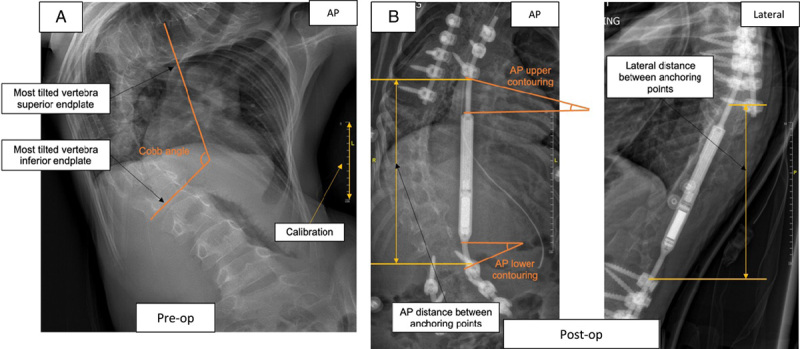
Imaging analysis measurements. A, Preoperative AP radiograph showing the preoperative Cobb angle measurement; (B) postoperative AP and lateral radiograph showing the postoperative measurements, comprising the distance between anchoring points, upper and lower rod contouring degree in the AP and lateral planes. AP indicates anteroposterior.
